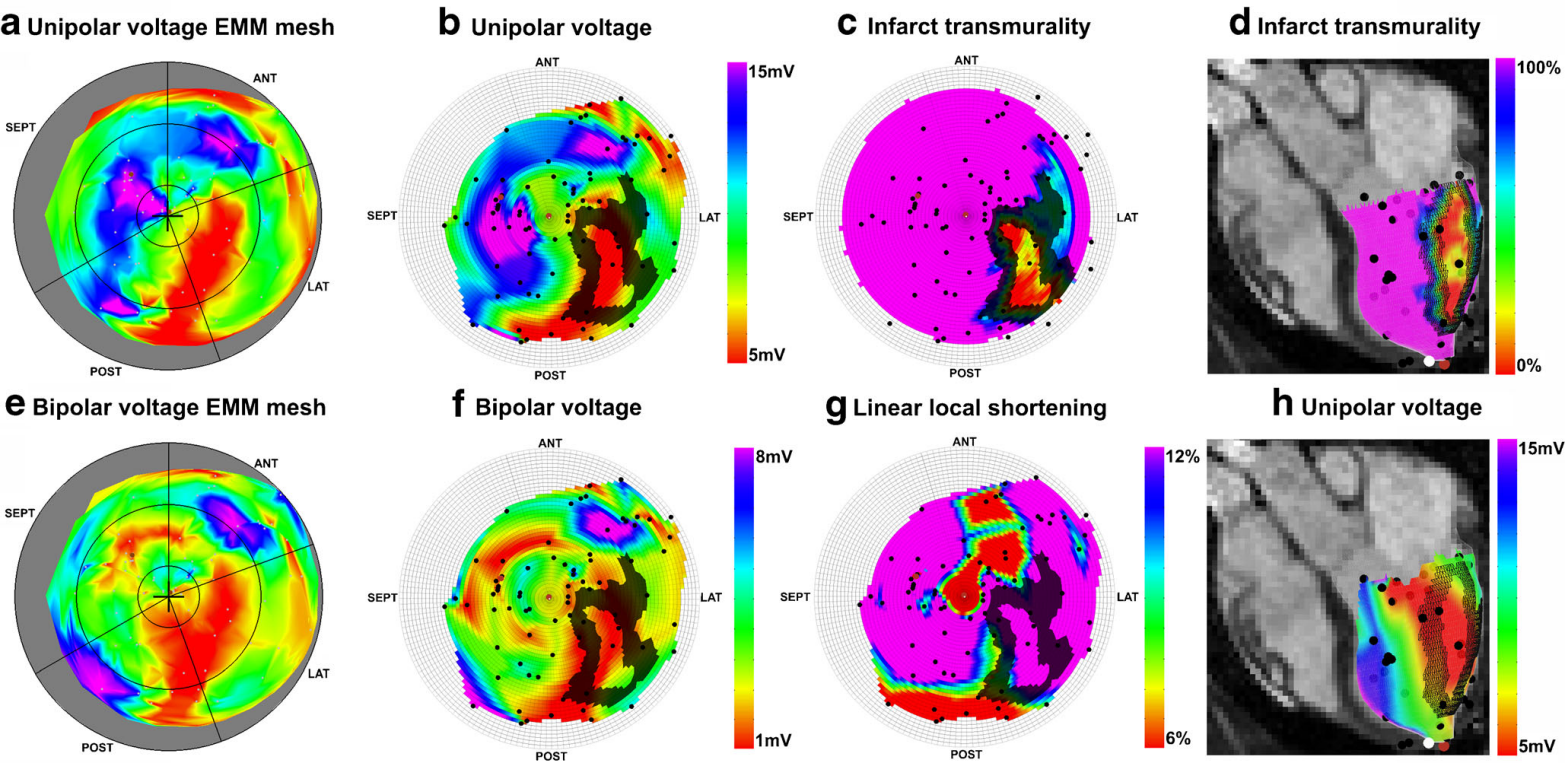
Typical bullseye plots of the NOGA system: unipolar voltage (a), bipolar voltage (e). And data projected on the cine mesh: unipolar voltage (b), bipolar voltage (f), infarct transmurality (c), linear local shortening (g). Infarct transmurality and unipolar voltages projected on the endocardial surface mesh placed in an end-diastolic long-axis cine MRI image (d and h). D and H represent the operator view during the image-guided injection procedure. Coloured dots are: NOGA measurement points (*black*), the apex (*white*), annotated location (*brown*). The *black* overlay in images b, c, d, f, g, h is a projection of the 50 % infarct transmurality border zone
